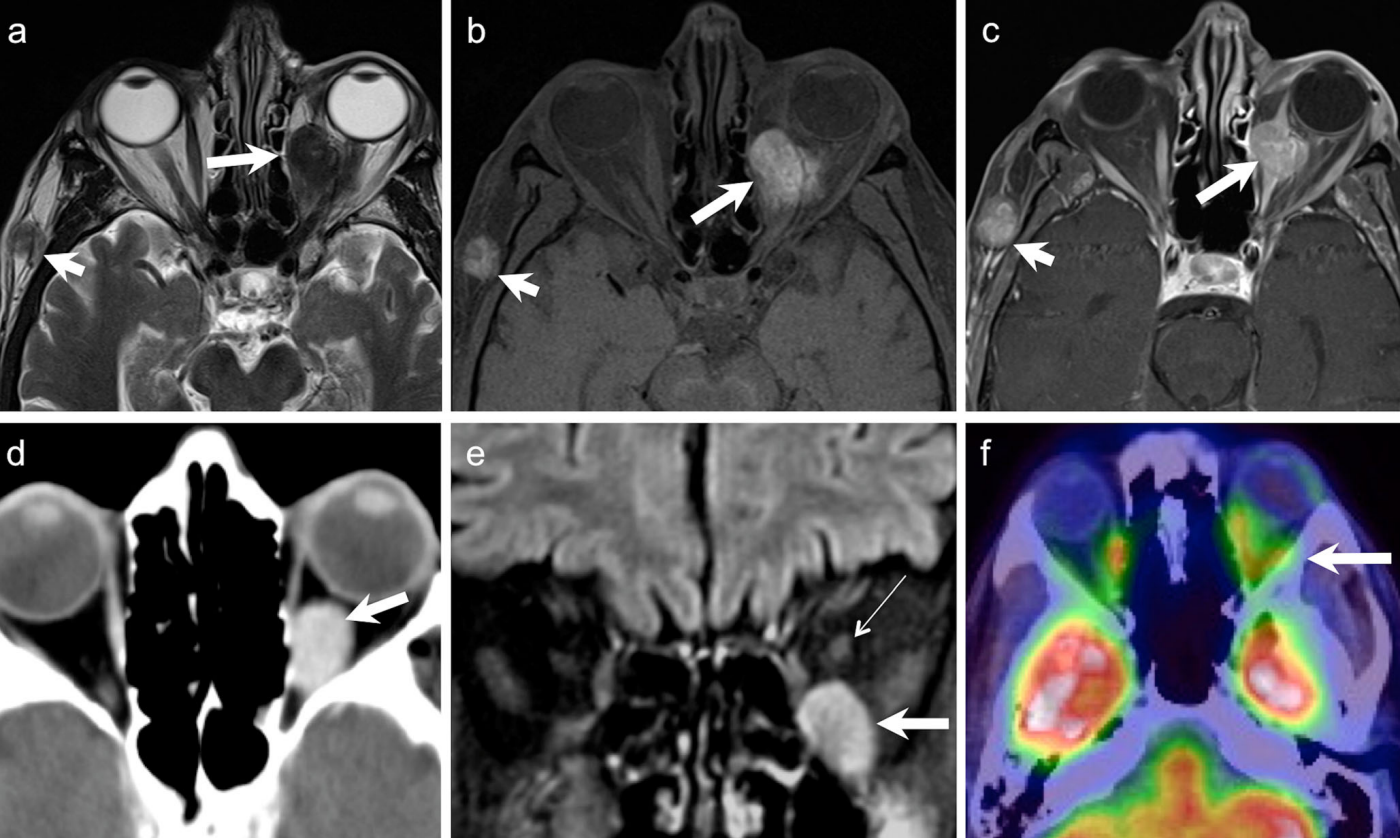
Two different patients with orbital metastases. a – c. 74-year-old female patient with diplopia and a history of melanoma of the scalp 7 years previously. T2W (a), unenhanced FS T1W (b) and contrast-enhanced FS T1W (c) images of the orbits shows a conal-extraconal mass in the left orbit (arrows) and a second mass with similar imaging features in the suprazygomatic right masticator space (short arrows). The strongly hyperintense signal in b (arrows) suggests the presence of haemorrhage and/or melanin. Imaging findings are strongly suggestive of metastases from melanoma. Findings were confirmed by biopsy. d – f. 71-year-old female patient with known breast cancer. d. Axial CECT image shows a well-circumscribed enhancing mass in the left orbit in extraconal location (arrow). e. Coronal STIR image of the same patient shows non-specific moderately high signal of the metastatic deposit (thick arrow). Thin arrow points to the left optic nerve. f. Axial PET/CT image of the same patient shows physiological high FDG uptake in the extra-ocular muscles making it difficult to detect the metastatic lesion (arrow). As the imaging findings in this case are non-specific, the diagnosis of orbital metastasis can be suggested only when the clinical background is known. Biopsy confirmed metastasis from breast cancer
